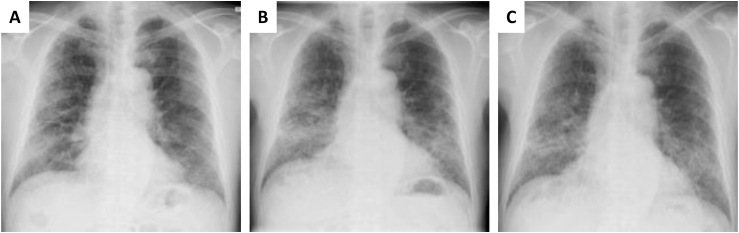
Serial changes of chest radiograph findings after the diagnosis of coronavirus disease 2019 (COVID-19). (A) At the diagnosis of COVID-19 (B) At the diagnosis of acute exacerbation of IPF (C) After the first methylprednisolone pulse therapy.
